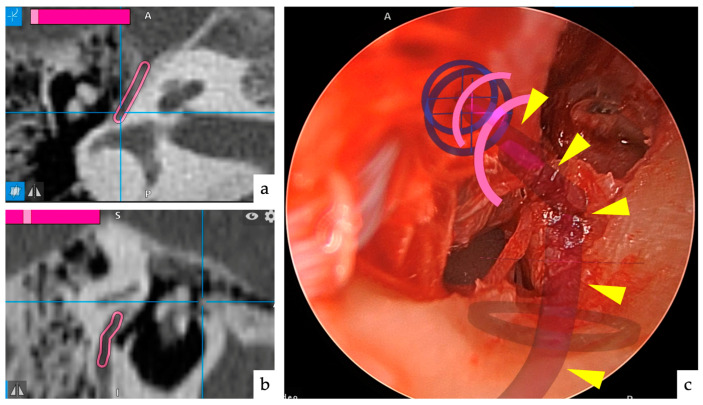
Augmented reality (AR) image of the facial nerve. (a) Marking the facial nerve with a pink line on the axial computed tomography (CT) slice of the temporal bone. (b) Marking the facial nerve with a pink line on the sagittal CT slice of the temporal bone. (c) An AR image of the facial nerve overlaid on the endoscopic image (yellow arrowhead tip). It is not a 3D display; therefore, its depth is not known, but its direction is indicated.
